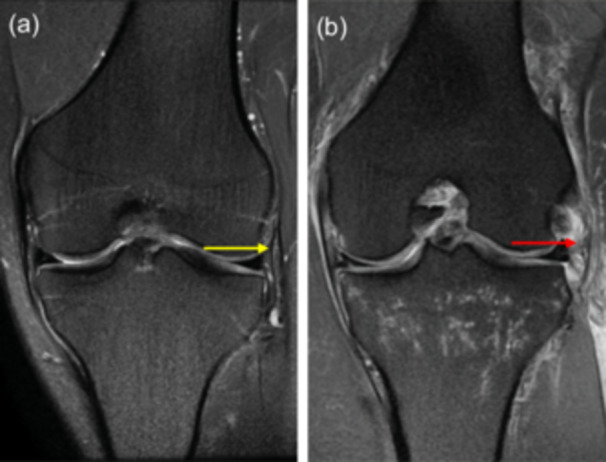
Magnetic resonance images demonstrating the intact (a) (yellow arrow) and abnormal (b) (red arrow) anterolateral ligament.
